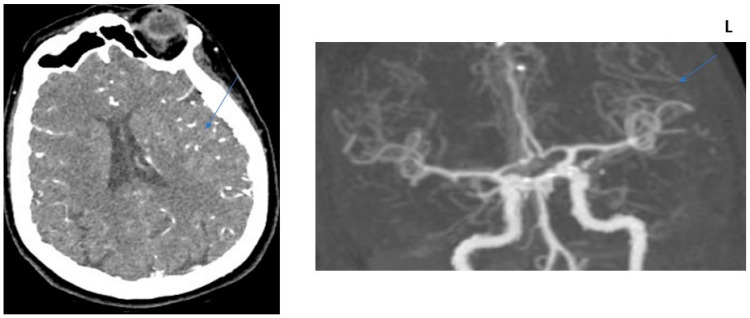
COLLATERAL SCORE: 3–75-year-old male patients. Left MCA infarction. NIHH Score: 9. CT angiography was performed 5 h after the acquisition of clinical findings. Left image, “Subtracted MIP image obtained from arterial phase images”. Right image, “Raw image in the axial plane obtained in the arterial phase after contrast injection”. Arrows represent collateral score area.
